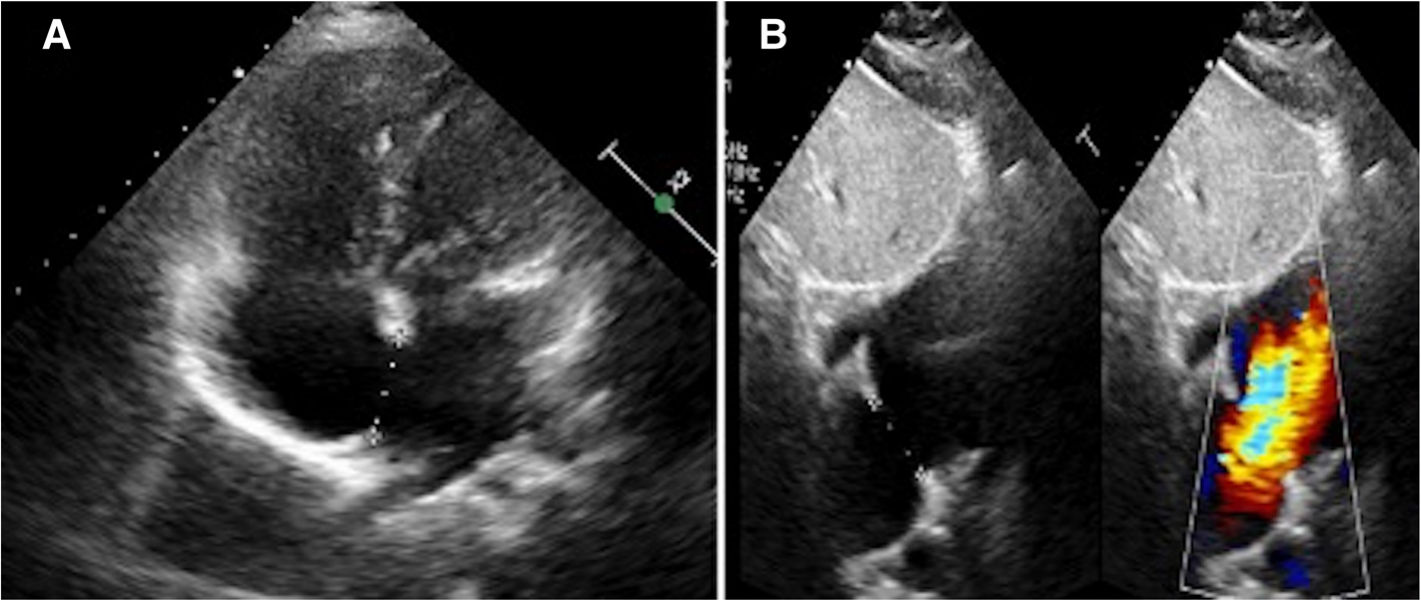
Transthoracic echocardiogram showing an 18-mm Secundum atrial septal defect with dilated Right atrium and right ventricle (A) and left-to-right flow across the defect (B)
